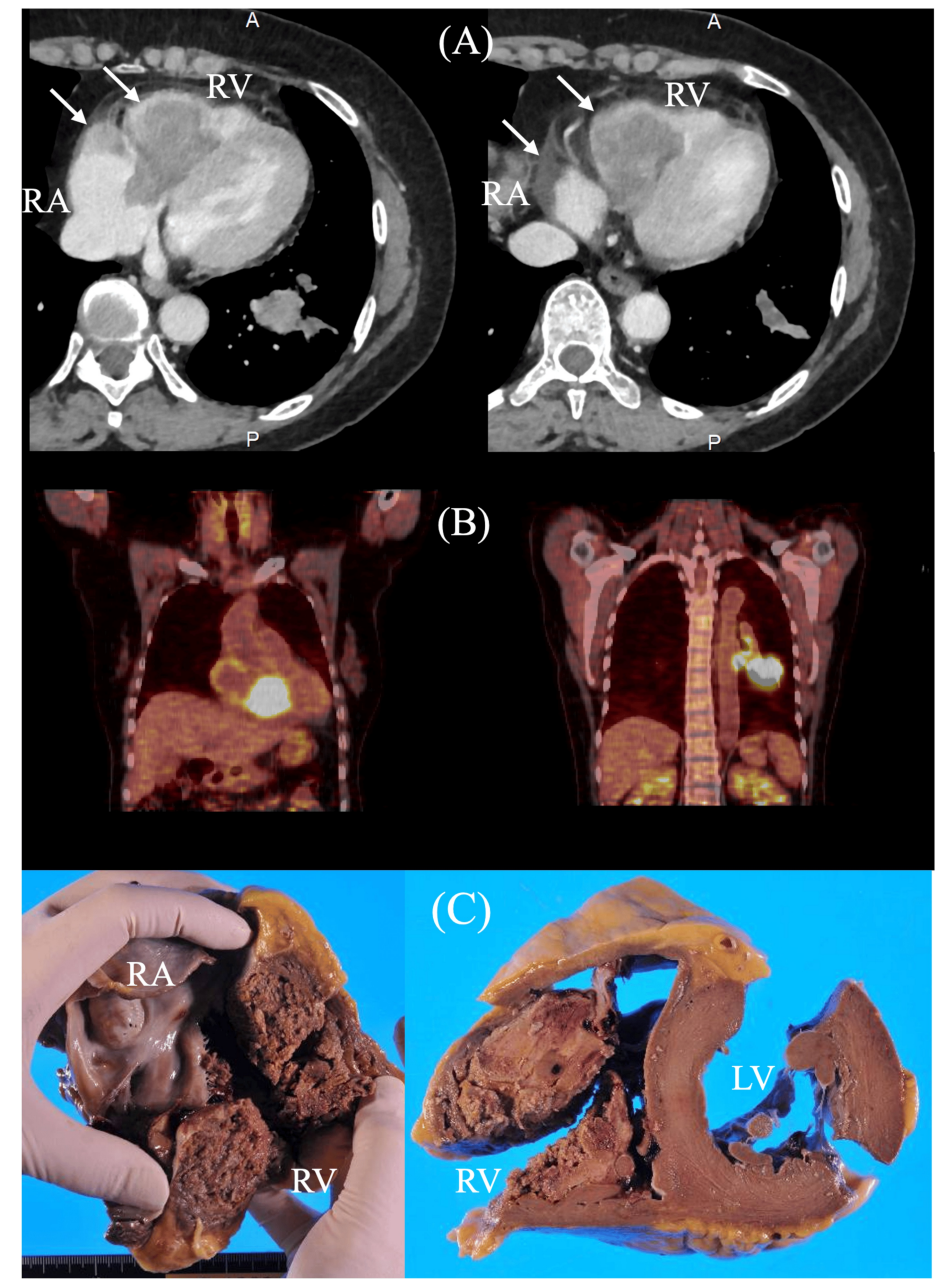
(A) Contrast-enhanced CT at initial presentation showing the right ventricle (RV), including the tricuspid annulus, completely occupied by a tumor mass (arrow). (B) PET scan revealing abnormal high uptake in the left lung, indicating distant metastasis. (C) Autopsy demonstrating massive tumor involvement throughout the right-sided cardiac chambers (Case 5). RV: right ventricle, RA: right atrium, CT: computed tomography, PET: positron emission tomography.
